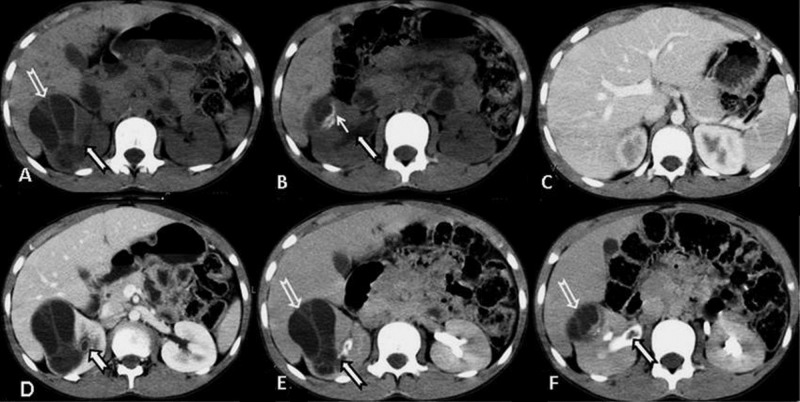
Hydatid cyst in the kidney on CT Axial unenhanced (A and B), nephrographic (C and D), and excretory phase (E and F) images from a CT urogram demonstrate a moderate-sized, non-enhancing, low-attenuation,well-marginated, multi-septated cystic mass in the right kidney involving the outer cortex anterior to the renal pelvis (open white arrow) with peripheral calcifications dorsally (thin white arrow). Extension of the daughter cysts is noted in the right pelvicalyceal system extending into the right ureter which is suggestive of the rupture of the parent cyst (white arrow with black outline). CT: computed tomography
